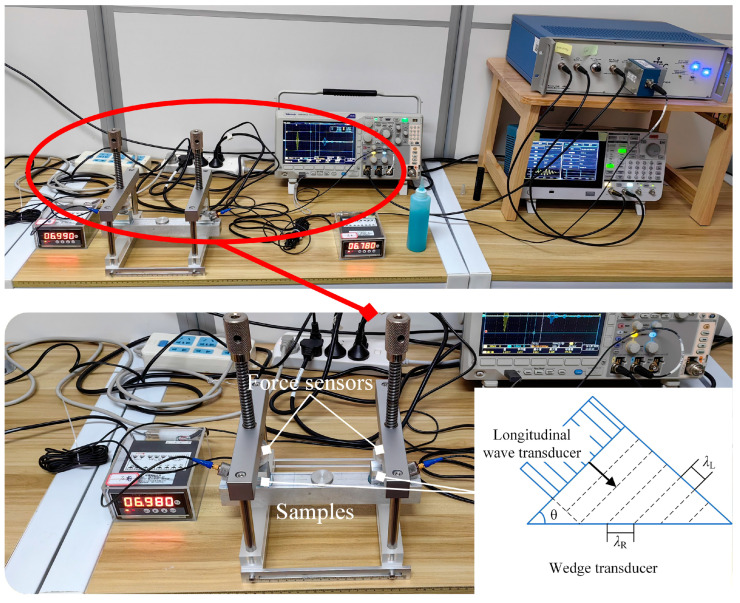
The ultrasonic measurement system.
